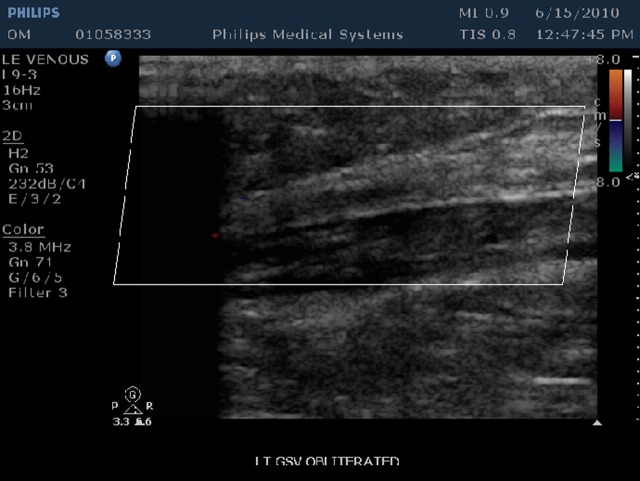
Ultrasound of closed GSV at 12 months (no flow and complete vein wall retraction). GSV, great saphenous vein
